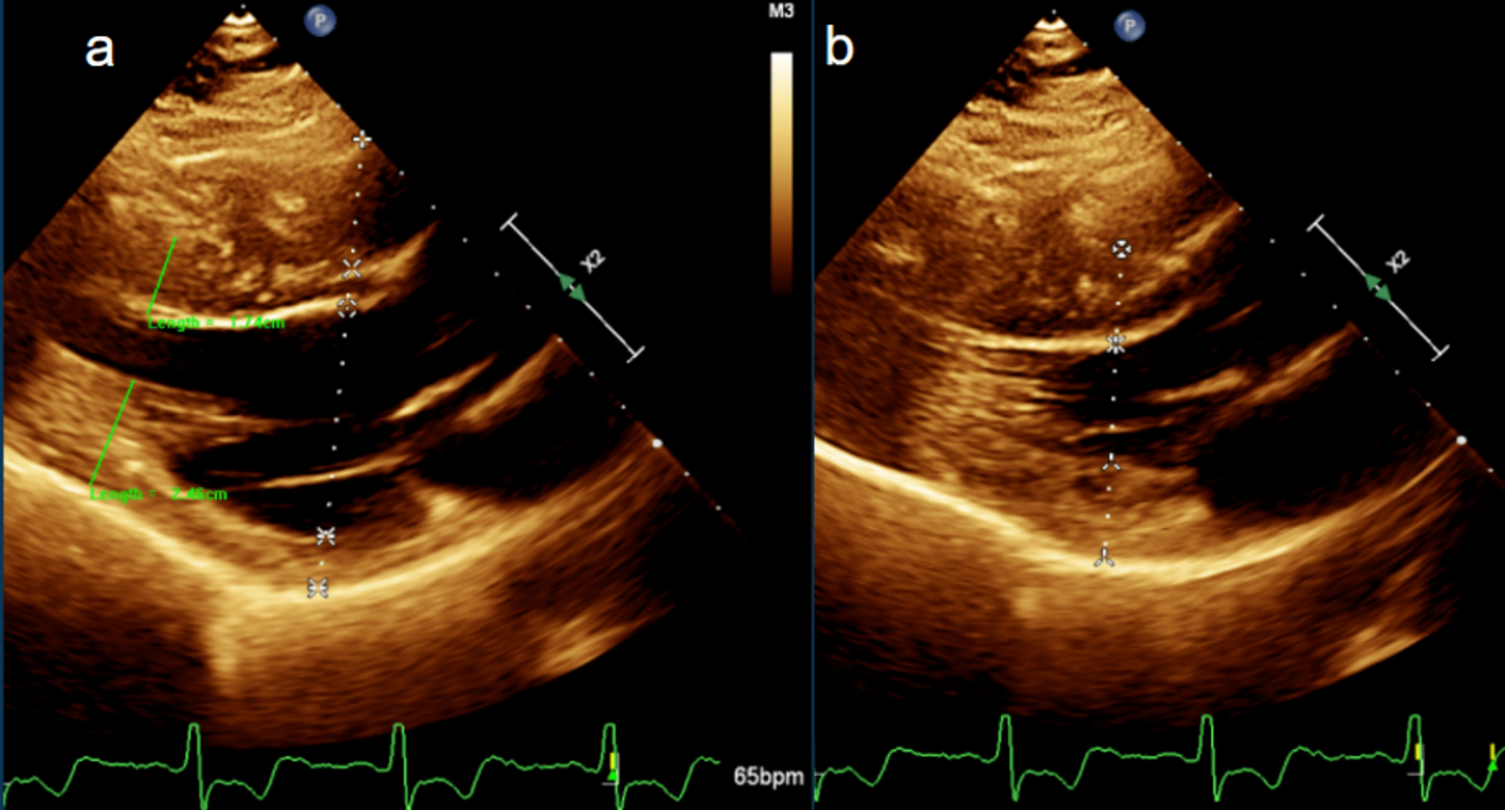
ApHCM transthoracic echocardiography parasternal long axis view in diastole (a) and in systole (b) showing maximal wall thickness of 17.4 mm. ApHCM: Apical hypertrophic cardiomyopathy
